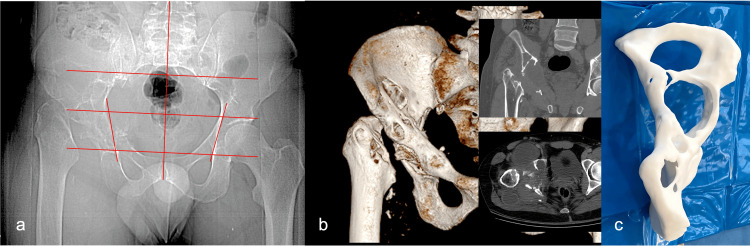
Clinical Case 2: preoperative imaging studies Initial X-ray evaluation (a); CT scan showing multiple collections and cysts (b); and hemipelvis PLA (polylactic acid) 3D-printed replica with evidence of severe bone loss (c).
